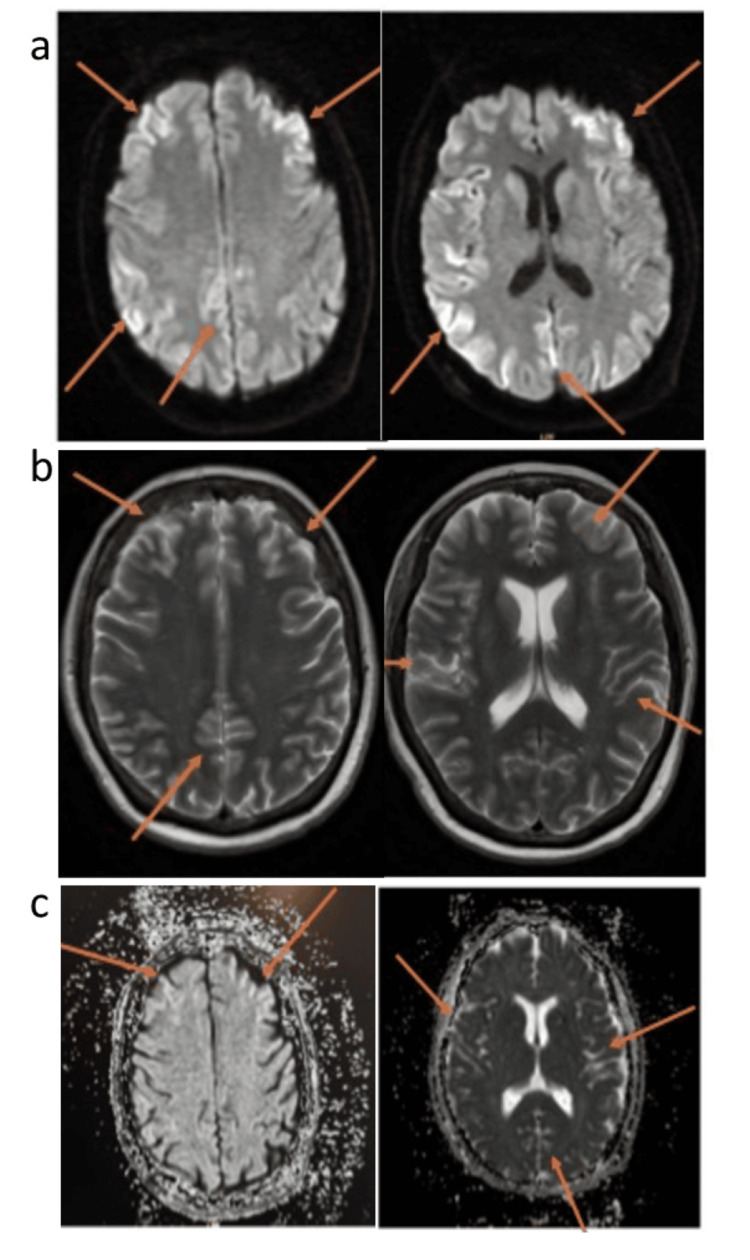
Bilateral cerebral hemispheres almost symmetrical cortical diffusion restriction, showing; a) high signal on DWI, b) low signal on ADC map and c) slightly increased signal on T2/FLAIR sequence DWI: diffusion-weighted imaging, T2/FLAIR: T2-weighted imaging/fluid-attenuated inversion recovery, ADC: apparent diffusion coefficient.
